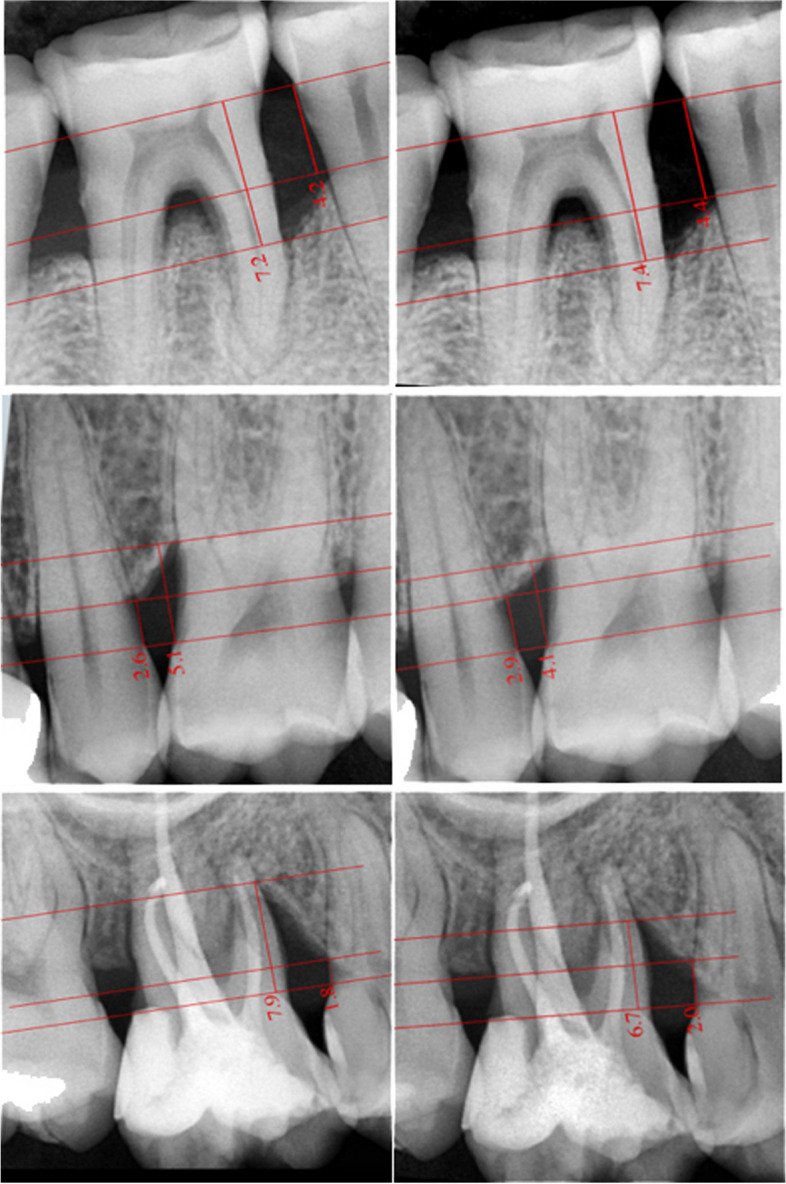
Radiographic views for the periodontal vertical defect at baseline (left) and at 6 months postoperative (right) for OFD (up left and right), L-PRF (middle left and right), and L-PRF + CM (below left and right)
